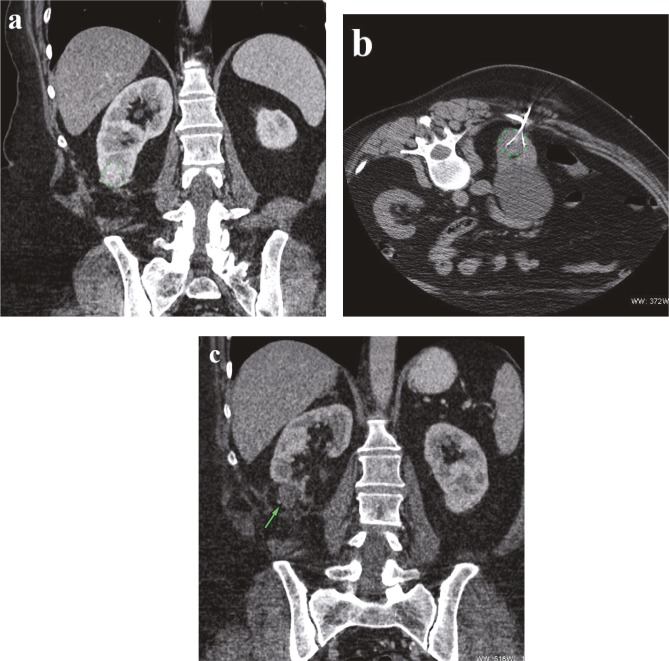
a) Fifty-one-year-old woman with 2.5-cm biopsy-proven renal cell carcinoma marked with green dots. RENAL nephrometry score calculated as 6. b) CT image obtained with patient in prone position at RFA shows needle electrodes in tumor marked with green dots. c) CT image obtained with IV contrast material shows mass is no longer enhanced after 3 years (green arrow).
